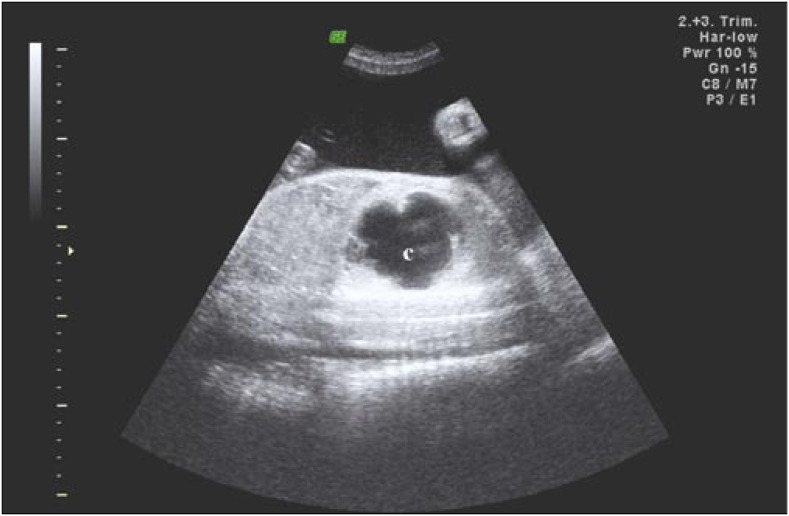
Sagittal section (two-dimensional ultrasound) through the left fetal thorax showing the hyperechogenic lung with a large cyst (c).
